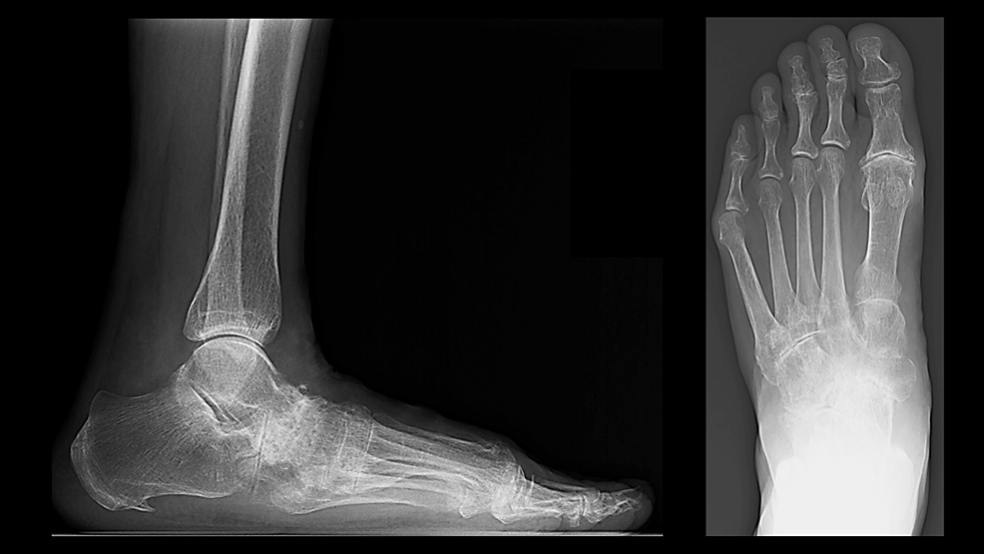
Preoperative radiograph of the foot in a weight-bearing position. The talonavicular joint is destroyed and shows end-stage arthritis. The talus shows a plantar flexion deformity.
